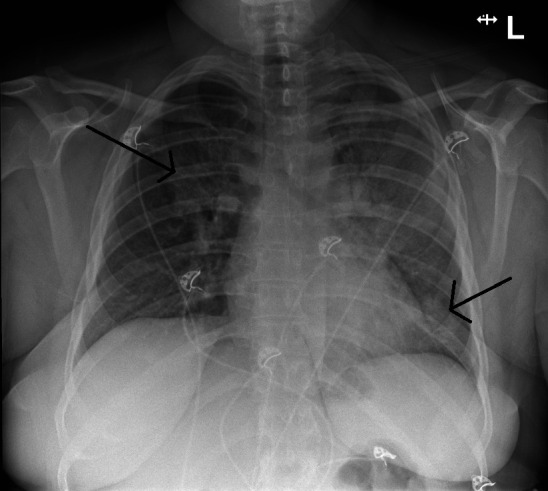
Posterior-anterior chest X-ray made during presentation at the emergency department showing consolidations in the left lobe and in the right upper lobe (indicated by arrows).
